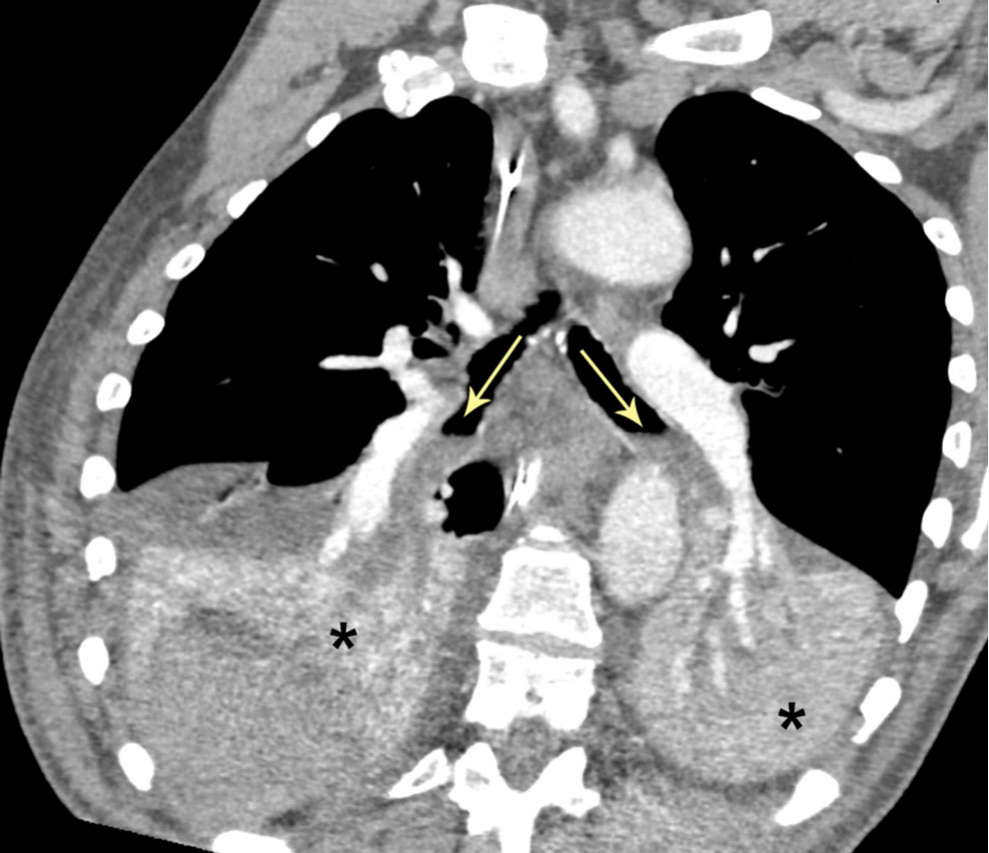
Bronchial obstruction due to acute aspiration. Double oblique CT image of an acute aspiration event. Note the layering low-density fluid in the bilateral lower lobe bronchi (*arrows*) causing complete collapse of the bilateral lower lobes (*)
